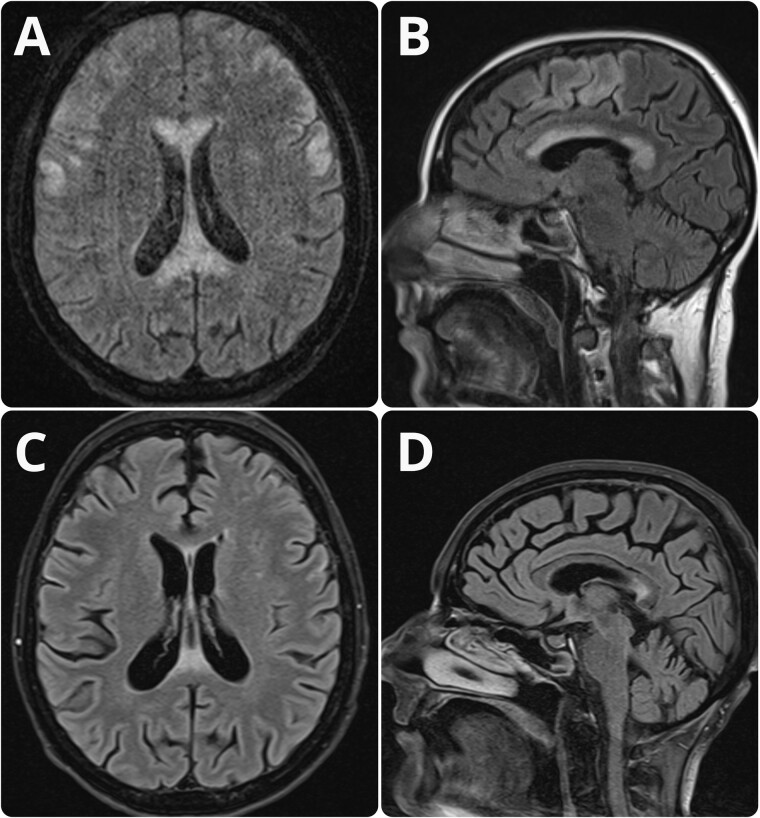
Significant decrease in the size of the primary area of involvement after 4 weeks of treatment compared with initial images. (A) and (B) T2-weighted MRI images showing the hyperintensity of the corpus callosum as well as the bilateral involvement of the cortical and subcortical regions of the frontal lobes. (C) and (D) T2-weighted MRI images revealing a significant decrease in the size of the primary area of involvement with residual hyperintensity of the splenium of the corpus callosum and complete regression of cortical and subcortical changes.
